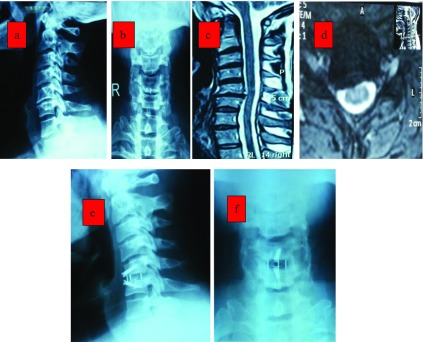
40 years old male with neck pain and right-sided radiculopathy of six months duration. X-rays (a, b) and MRI (c, d) revealed C5–6 prolapsed disc abutting the right exiting C6 nerve root. Follow-up X-ray at 12 months (e, f) showing fusion and maintained disc height.
